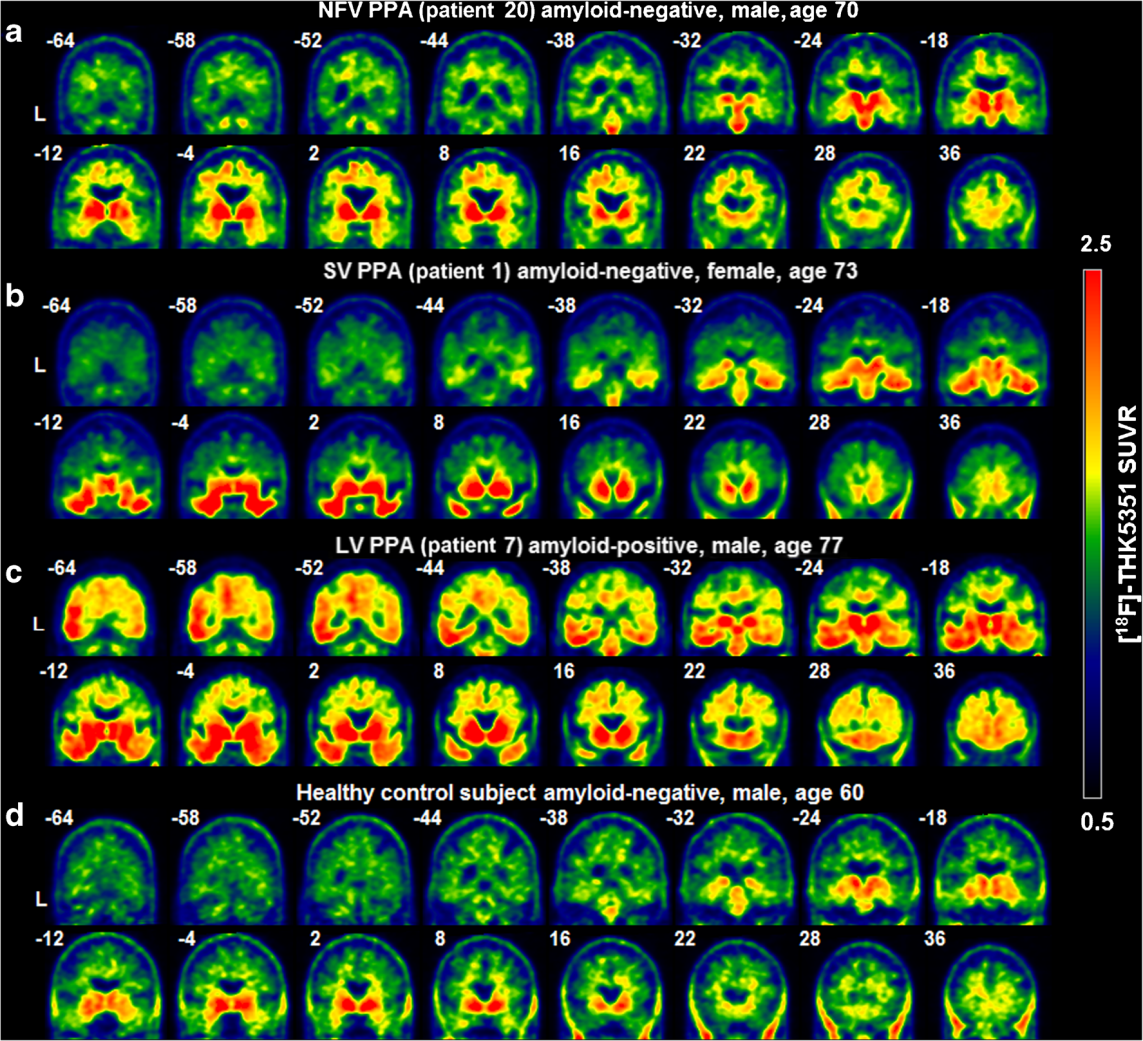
[^18^F]THK5351 SUVR images in representative individuals: a nonfluent variant (NFV) patient, b semantic variant (SV) patient, c logopenic (LV) patient, and d healthy control subject. The non-partial volume corrected SUVR intensity level is shown between 0.5 and 2.5. Patient numbers refer to Table 1
